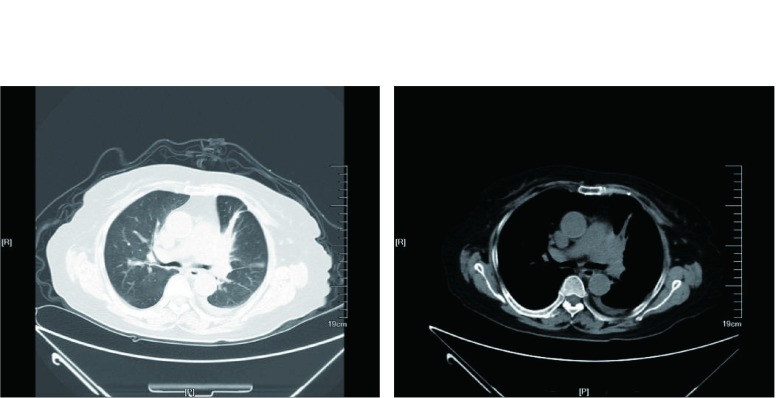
左主支气管前占位病变，与2015-11-13胸部CT比较变化不明显，伴有阻塞性肺炎（2015-12-23）。 Placeholder lesions before the left main bronchusis visible, no obvious changecompared with the chest CT of 2015-11-13, accompanied with obstructive pneumonia (2015-12-23).
